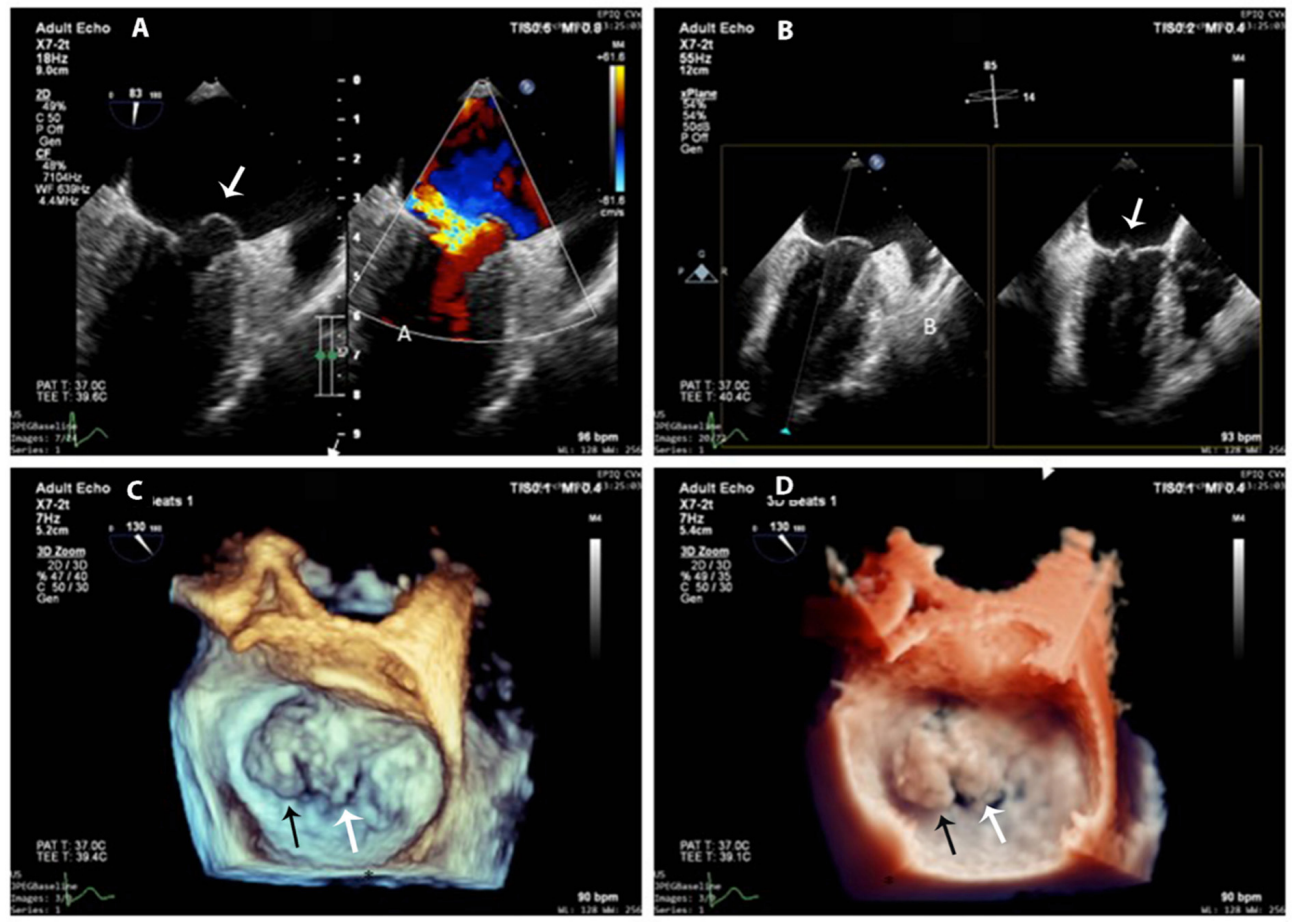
3D-TEE at 1-year follow-up. (A) Mitral anterior leaflet prolapse (white arrow) on a mid-esophageal two-chamber view (left panel) and the origin of the regurgitation jet with color Doppler (right panel). (B) X-plane imaging with two orthogonal views shows exactly the prolapsed scallops (white arrow). (C,D) 3D-zoom images of the mitral valve from the left atrial perspective (surgeon's en-face view) show exactly A1 (black arrow) and A2 (white arrow) prolapse (C) normal 3D gain vs. (D) true view mode.
